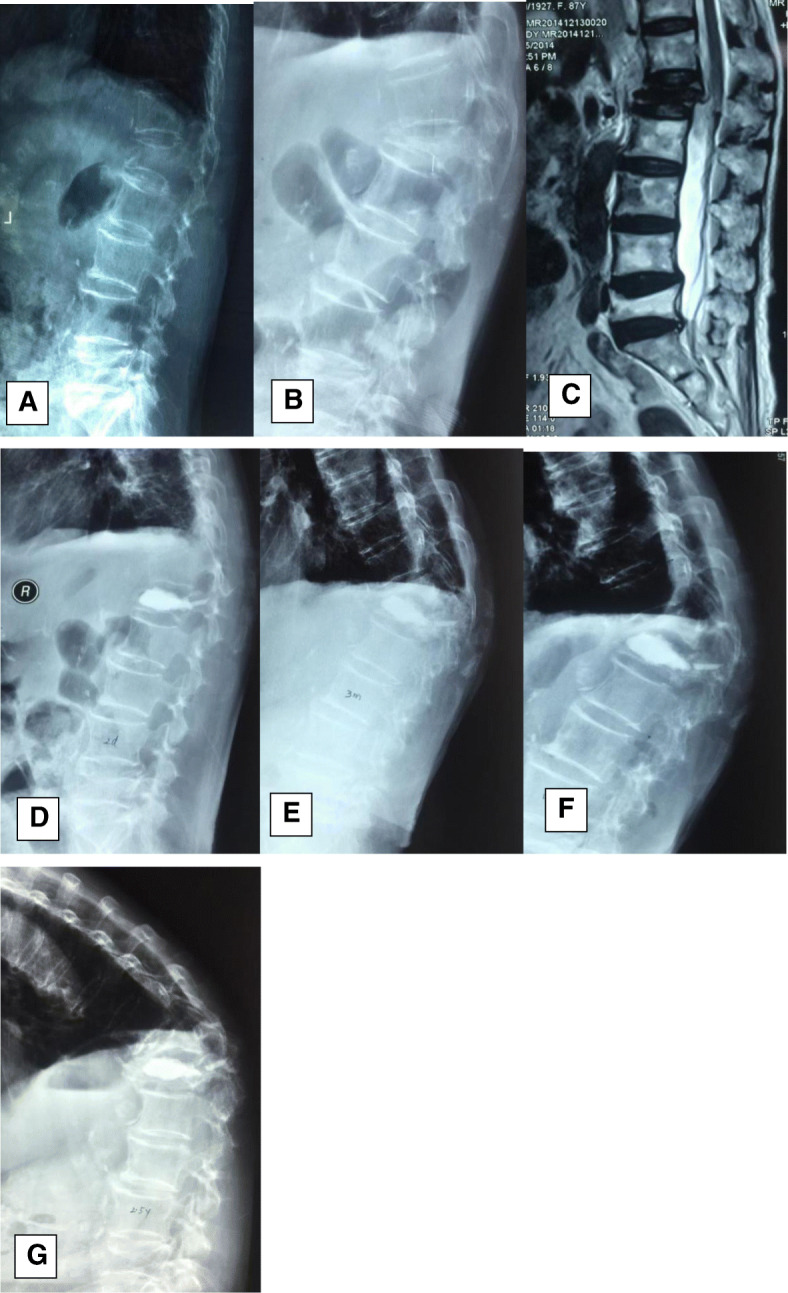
An 87-year-old female patient with an L1 osteoporotic fracture; conservative treatment failed, and then she was treated surgically with PV. One month after the operation, an adjacent-segment fracture occurred. a On the injured day, the patient accepted conservative treatment. bc The first admission X-ray and MRI showed a lumbar compression fracture at L1, and she underwent PV treatment. defg At the follow-up times of 3 months, 1 year, and 2.5 years postoperatively, X-ray showed vertebral collapse at L1, and a new adjacent-segment fracture occurred at T12; the kyphosis deformity deteriorated. However, the patient refused surgery, and she suffered from chronic back pain
